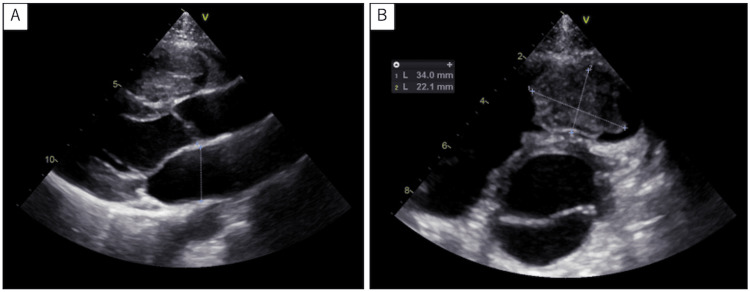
Echocardiographic view of the tumor in right ventricular outflow tract A. Parasternal long-axis tomogram and echocardiographic view of the tumor in the right ventricular outflow tract; B. Short-axis tomogram of aortic valve level and echocardiographic view of the tumor in the right ventricular outflow tract
